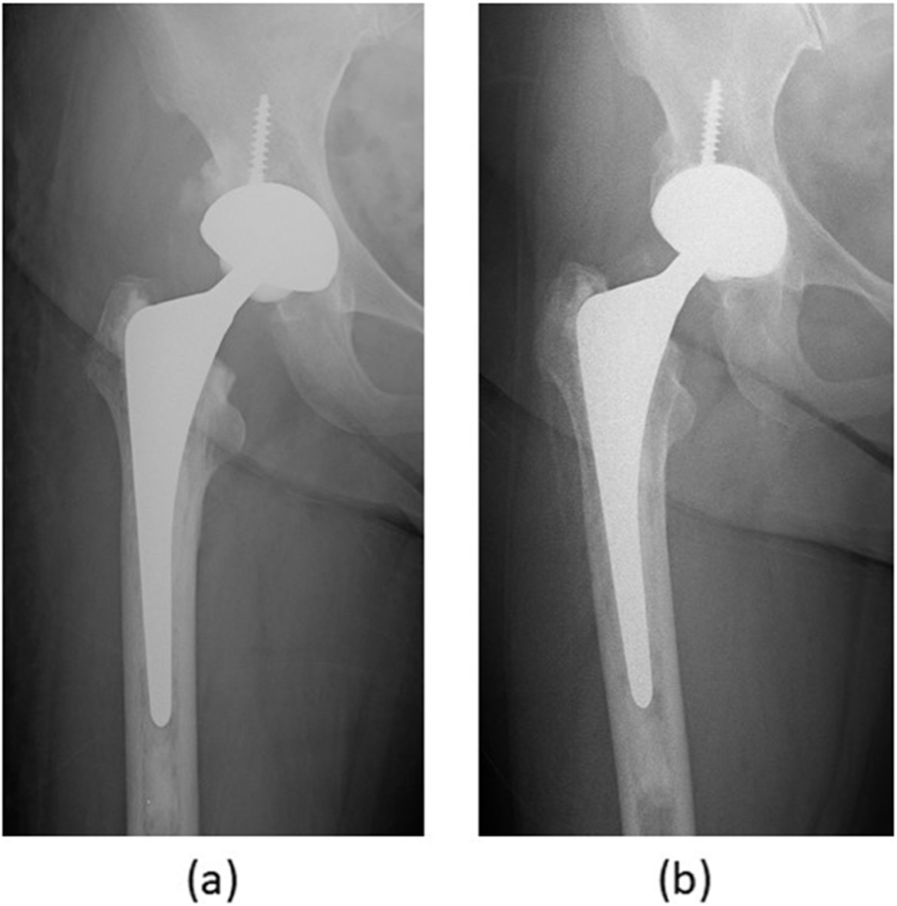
Post-operative anteroposterior radiographs of 62-year-old woman with dysplastic hip osteoarthritis showing (a) well-aligned stem cemented with line-to-line technique, (b) follow-up at 10 years without measurable subsidence of the stem
